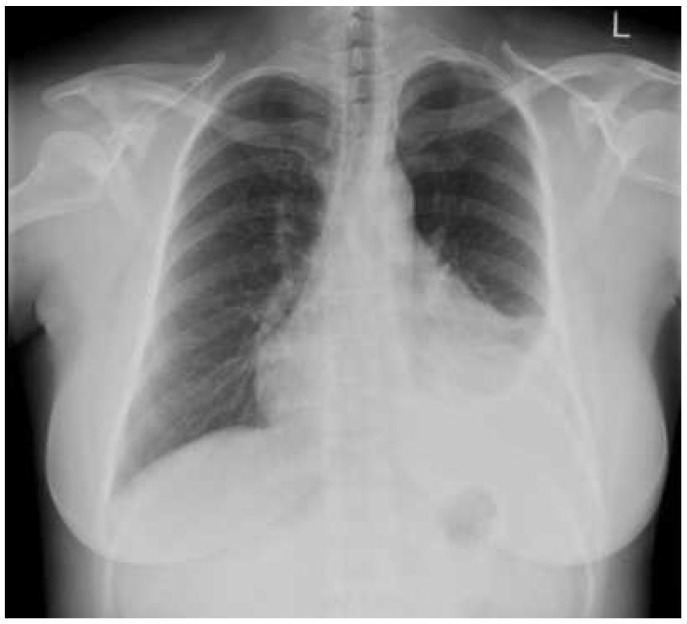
Chest radiograph (CXR) demonstrating a pleural effusion.
